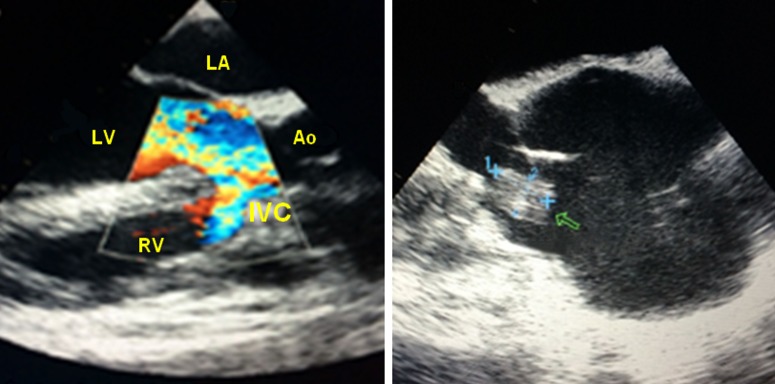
Transesophageal echocardiogram showing aortic regurgitation towards the right ventricle through an interventricular communication, and aortic valve vegetation (arrow). Ao: Aorta; IVC: Interventricular communication; LA: Left atrium; LV: Left ventricle; RV: Right ventricle.
